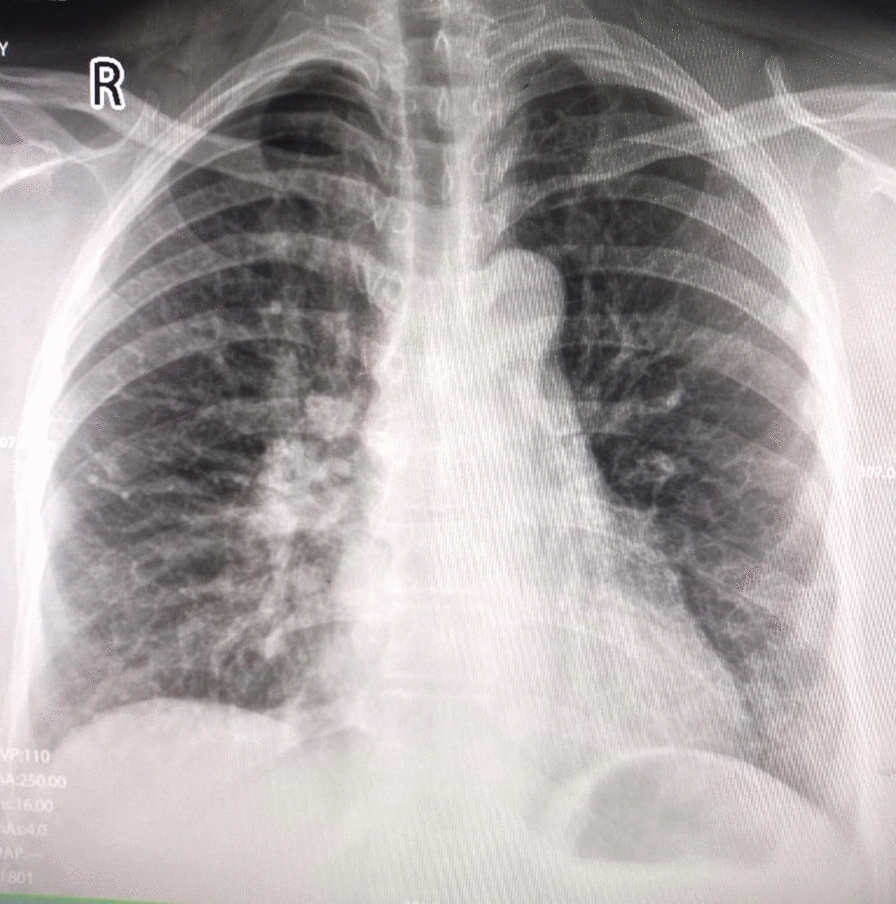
Posteroanterior view of chest X-ray showing left upper lobe hyperlucency and ipsilateral small hila
